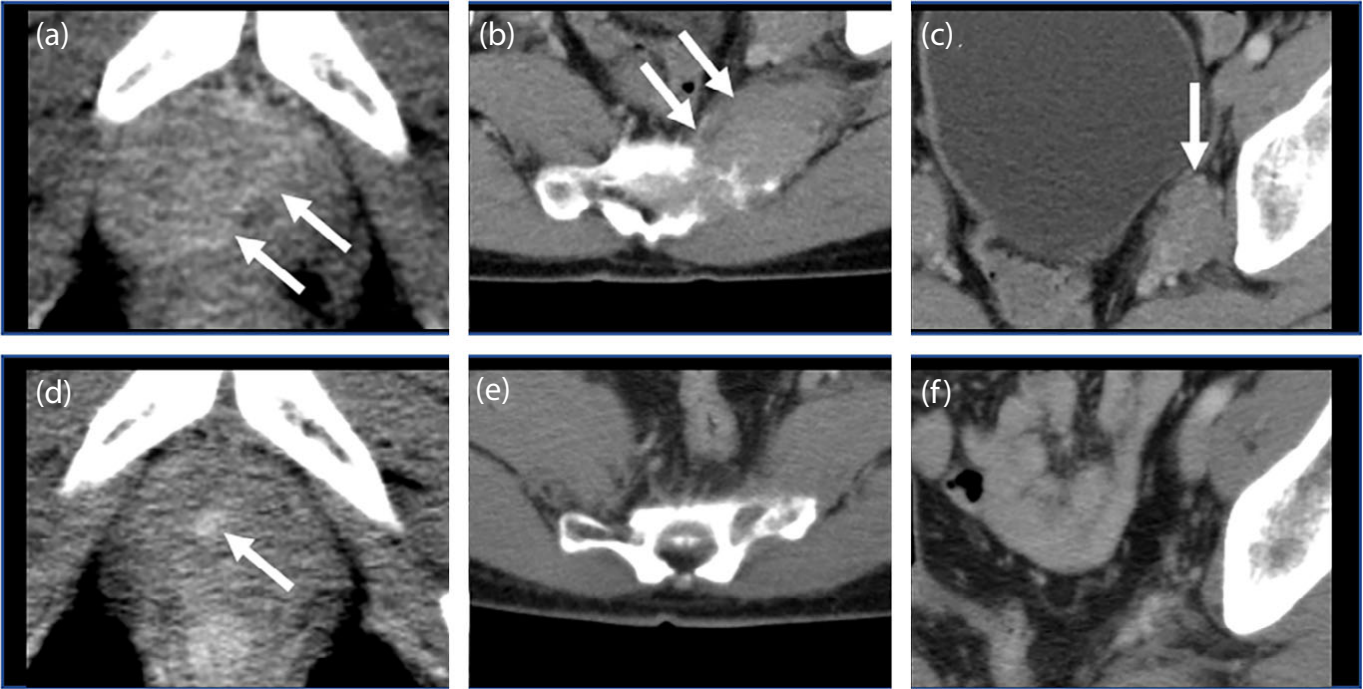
Pelvic CT before (a–c) and after (d–f) radiation therapy. Local recurrence on the dorsal pubis (a, d). Bone metastasis on the left side of the sacrum. (b, e). Left closed lymph node metastasis (c, f).
